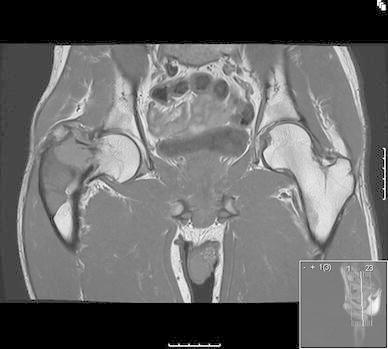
MRI scan of the pelvis demonstrating the fibrous dysplasia of the right proximal femur with the femoral neck fracture
